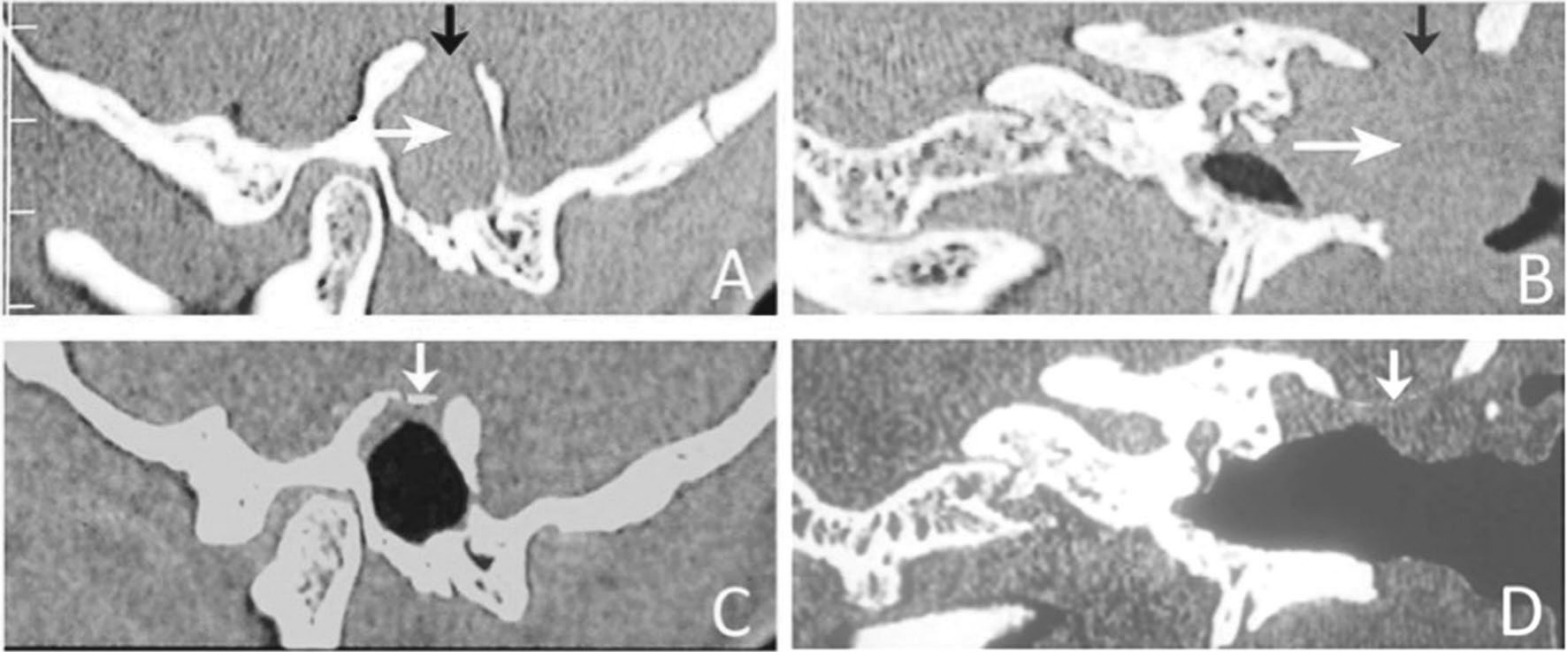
Preoperative (a, b) and 1-year postoperative (c, d) coronal and parasagittal computed tomography scan images passing through the skull base defect. a, b Note the large skull base defect (black arrow) and a large herniation of the cranial contents (white arrow) in the external auditory canal and the open mastoid cavity. c, d Note the clearly defined bone shadow (white arrow) of the unit-sandwich graft. The bone graft has maintained its original intended position
